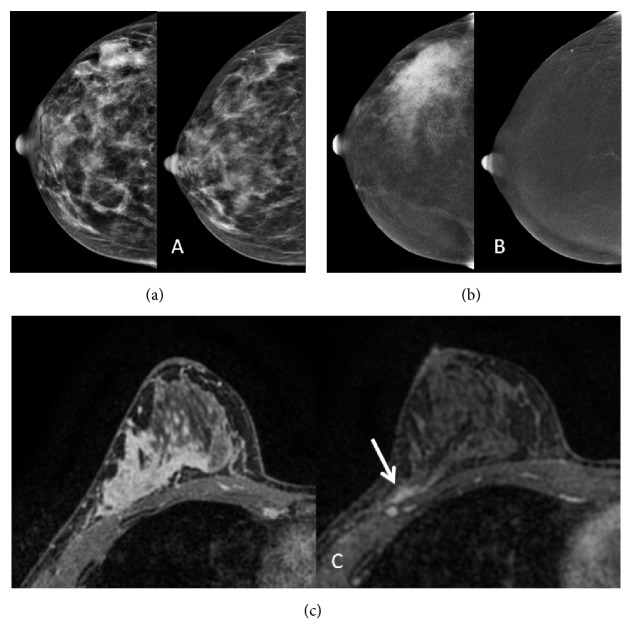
39-year-old woman. Invasive ductal carcinoma with pathologic complete response. Pre- and post-NAC FFDM (a), CEM (b), and MRI (c). Focal asymmetry is seen in the outer quadrant in FFDM (a). Pre-NAC CEM (b) and MRI (c) show segmental non-mass enhancement. Post-NAC FFDM (a) and MRI (c) incorrectly showed residual disease (arrow) while CEM was truly negative.
